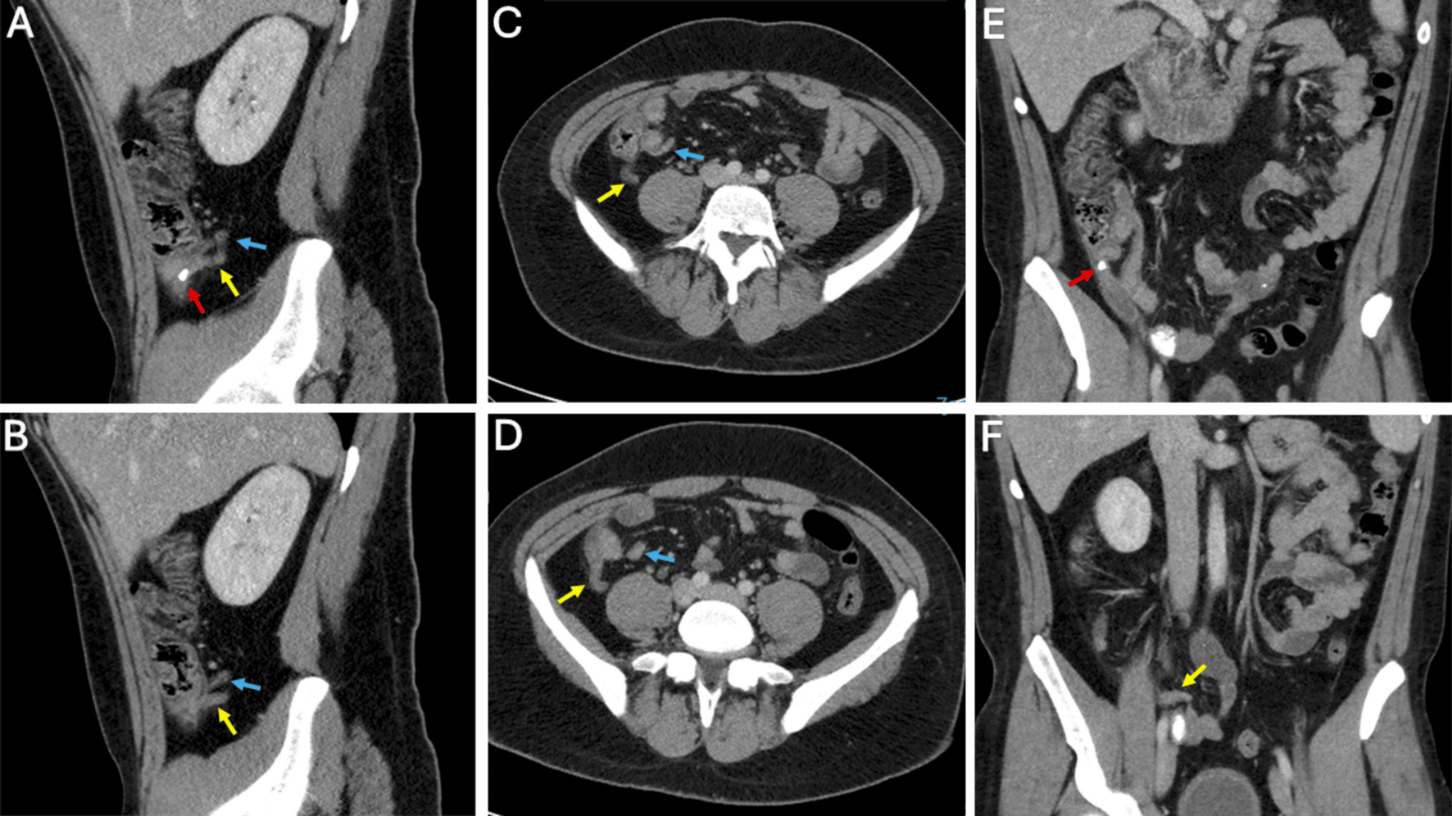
Contrast-enhanced computed tomography scan revealing a thickened and dilated appendix (yellow arrow) with appendicolith (red arrow), as well as a second appendix (blue arrow) in a type B2 configuration. Sagittal (A, B), axial (C, D), and coronal (E, F) views are shown.
